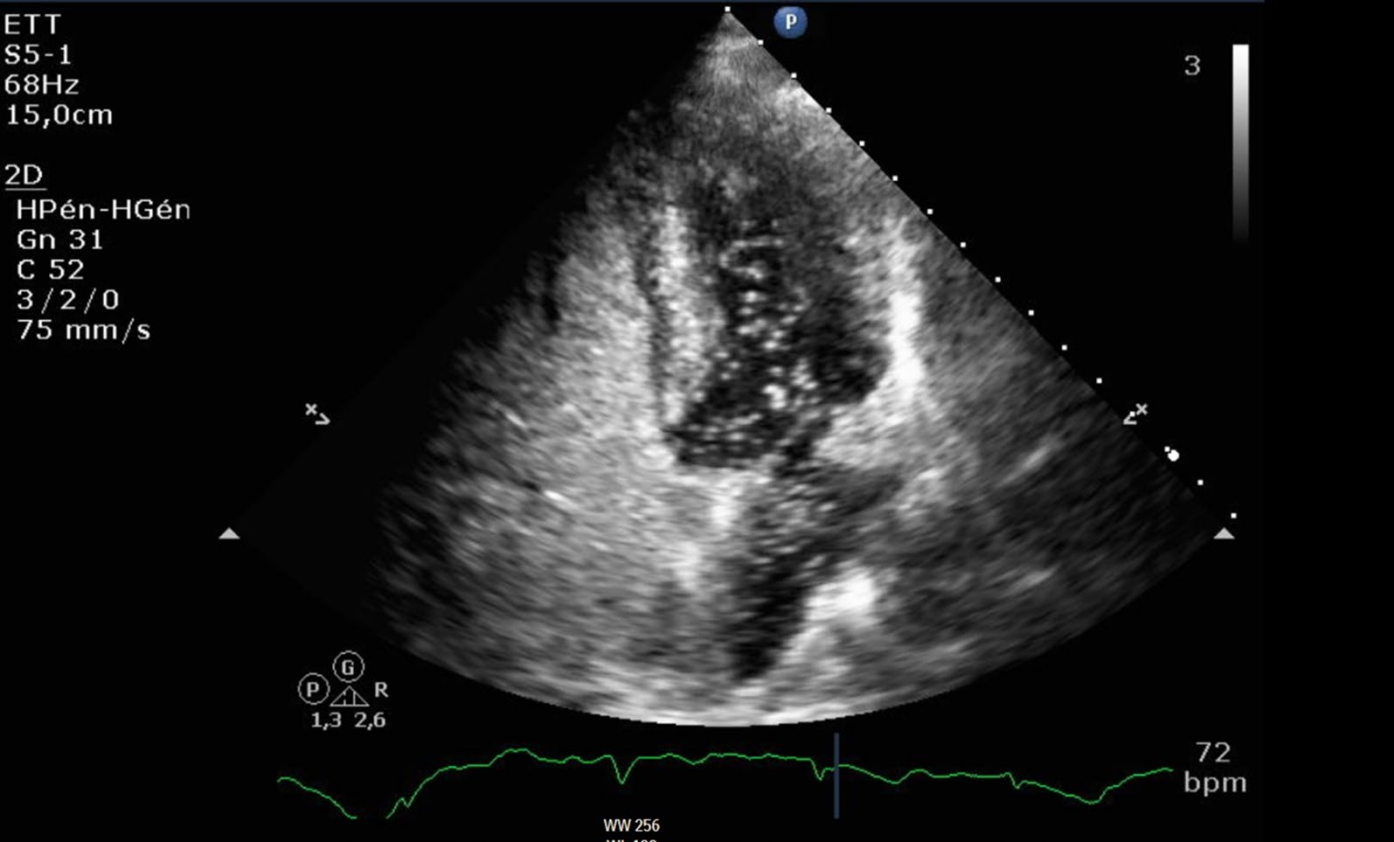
Bubble contrast echocardiography. Two-dimensional transthoracic echocardiography image showing a right-to-left shunt upon release of the Valsalva maneuver
